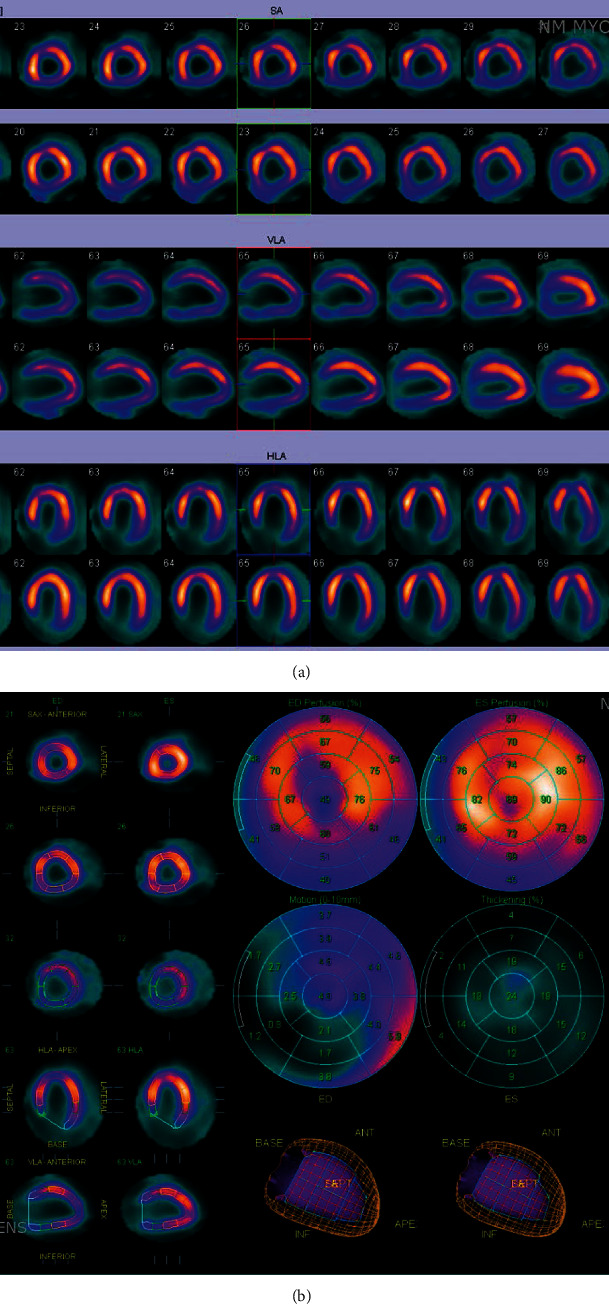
(a, b) The patient achieved 11 METS on a modified Bruce protocol but developed symptomatic chest discomfort and inferior lead ST-segment depressions at peak exercise persisting 1 minute into recovery. SPECT images demonstrated a reversible inferior wall perfusion deficit.
